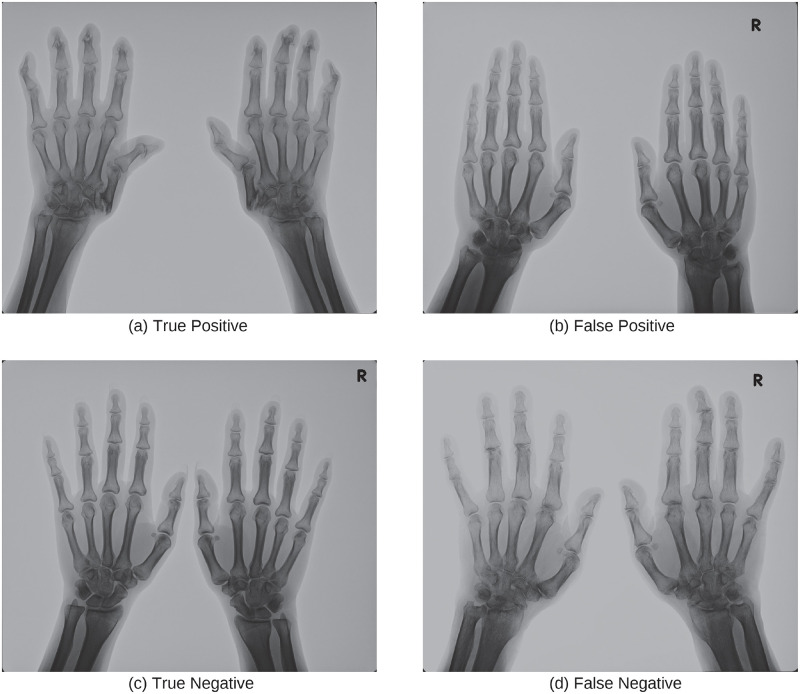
Examples of X-ray images in subluxation dataset. The caption of each image indicates the prediction result.
